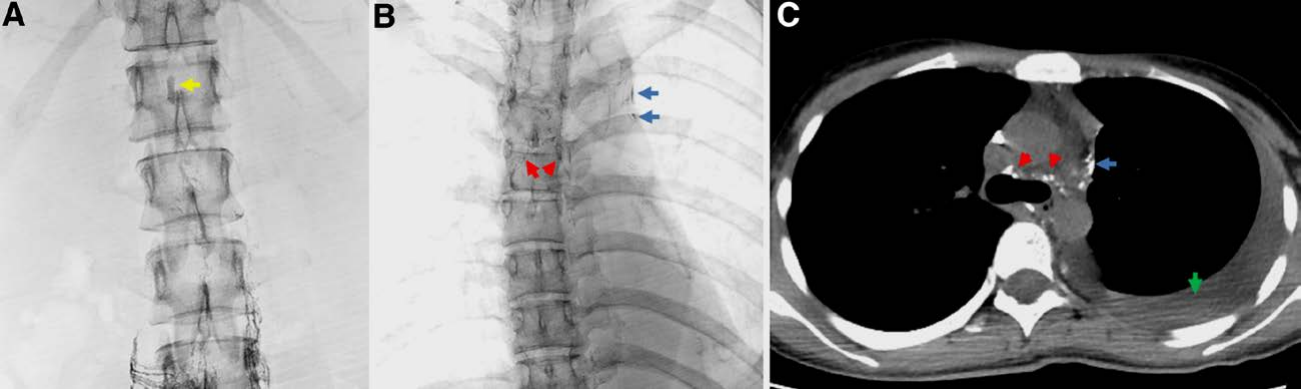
Lipiodol-based lymphangiography and CT showing the lymphatic plexus instead of the thoracic duct and leakage from the vessels. Lymphangiography under fluoroscopy showed a capillary network instead of the thoracic duct and leakage on the left side of the middle mediastinum. (A) Abdominal lymphatic channels showed a chylous cistern without thoracic duct. (B) thoracic lymphatic channels revealed the lymphatic plexus instead of the thoracic duct. (C) CT image at the chylous leakage level. (yellow arrow: chylous cistern; red arrow: plexus thoracic duct; blue arrow: leakage site; green arrow: chylothorax). CT = computed tomography.
